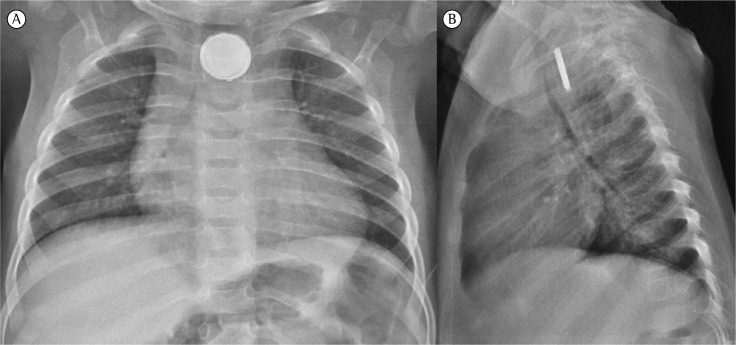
In A, an anteroposterior chest X-ray revealing a circular radiopaque shadow
